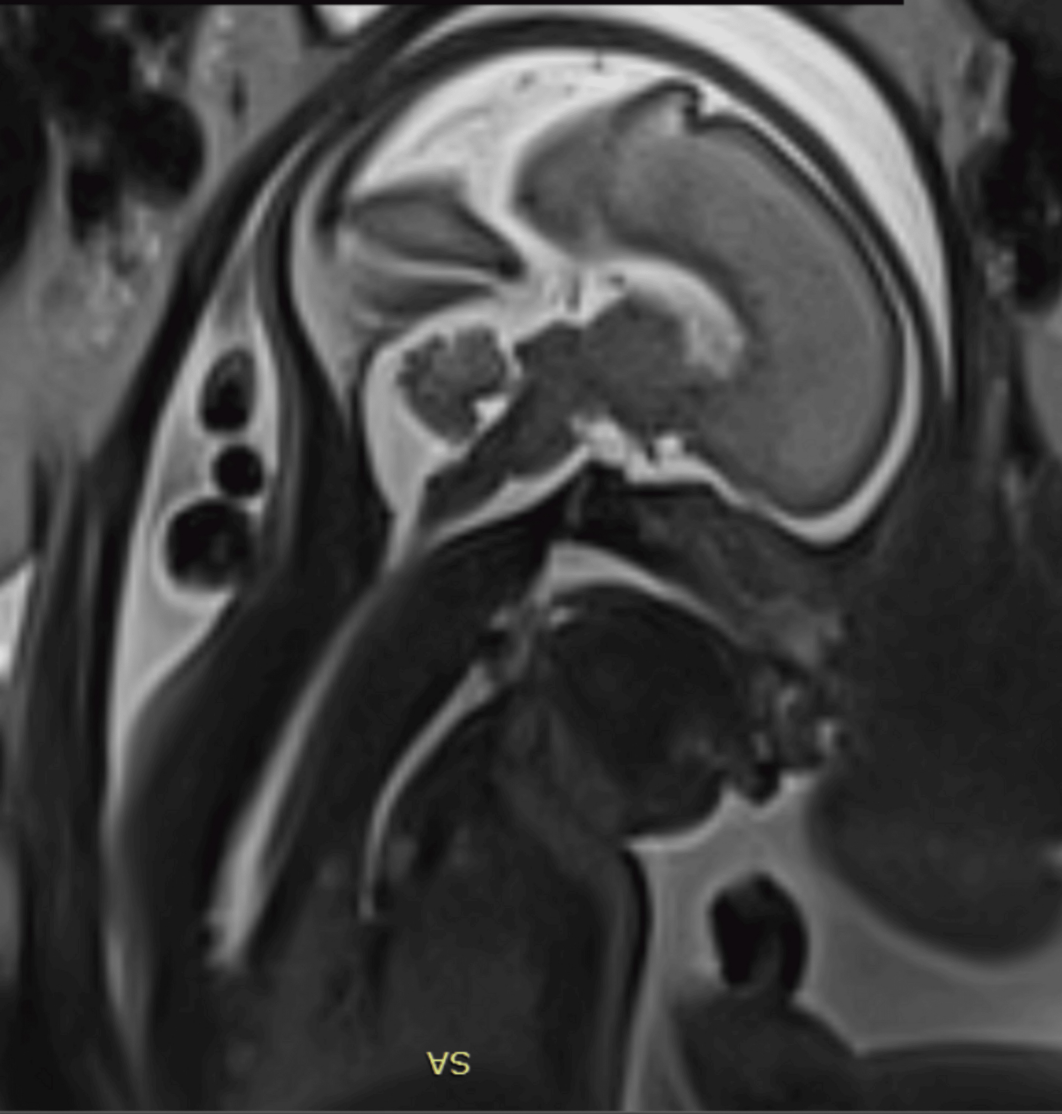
Fetal MRI: T2 sequence showing the absence of the corpus callosum
